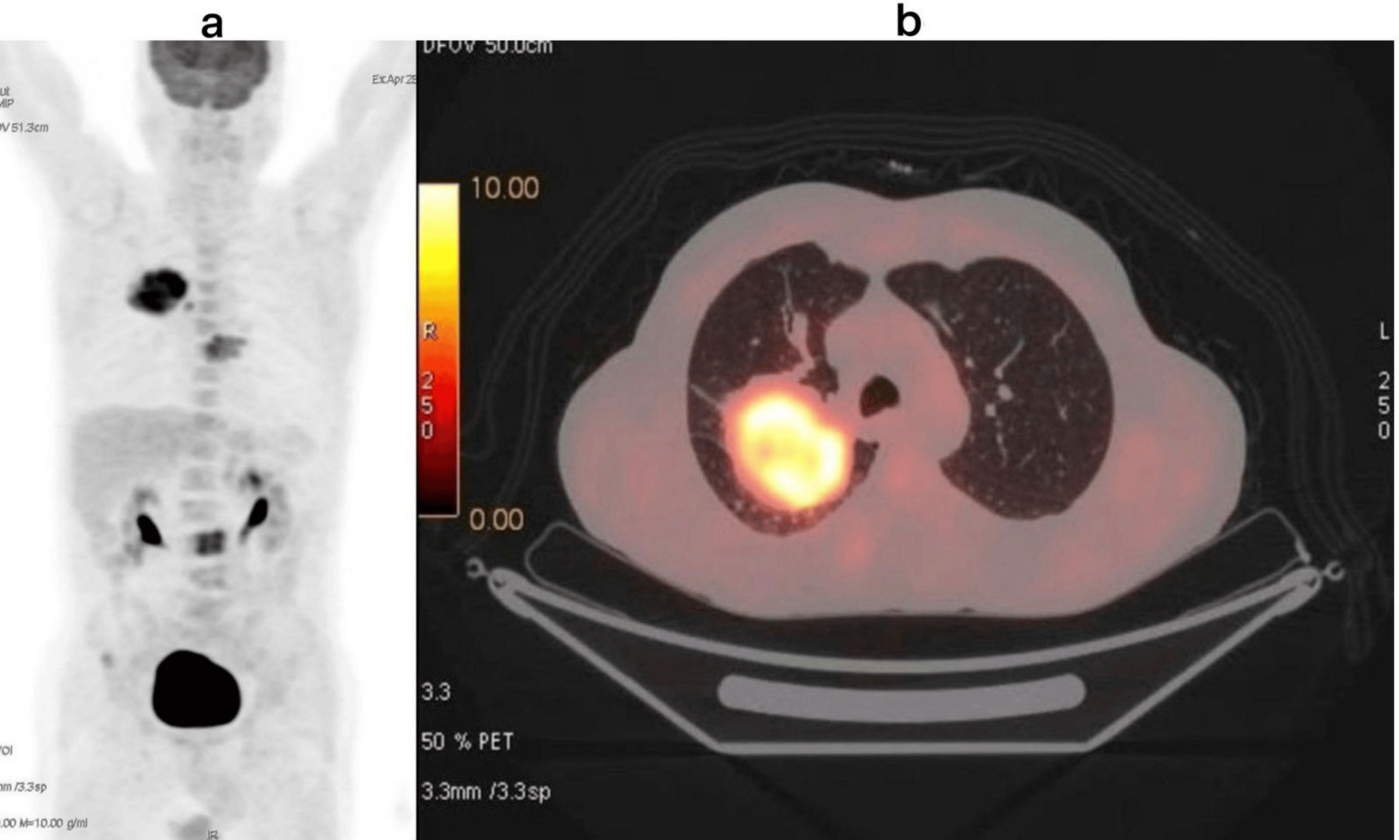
Images from the initial FDG-PET/CT showing the primary lung lesion (a: coronal view; b: axial view) FDG: fluorine-18 fluorodeoxyglucose
